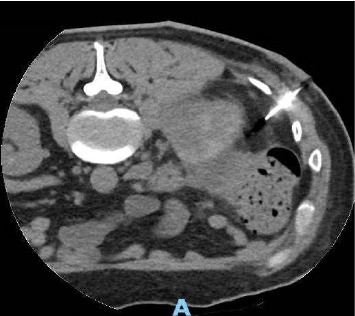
CT scan guided needle drainage of the perinephric abscess.
